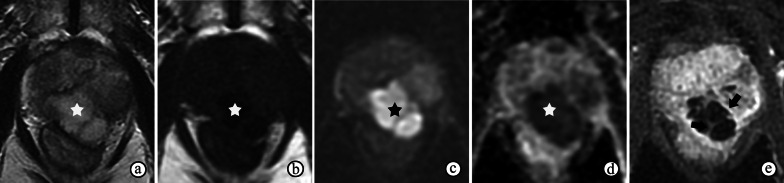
Prostate abscess in a 68-year-old male with dysuria for 2 months and a serum PSA level of 9.50 ng/mL, 16.9 white blood cells/high power field in urine and 1.7 epithelial cells /high power field in urine. a, b T2- and T1-weighted imaging show a mass with high T2 signal intensity and low T1 signal intensity (☆) in the PZ compressing the rectum. c, d The interior of the mass shows a very high signal intensity on DWI at a b-value of 800 s/mm^2^ and a very low signal intensity on the ADC maps (☆). e DCE imaging shows enhancement of the rim and separations in the middle (arrow)
